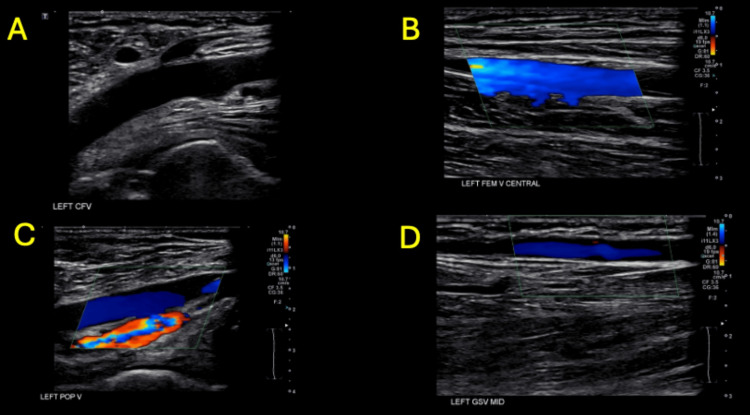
Left lower extremity venous duplex ultrasound There is no evidence of deep vein thrombosis or venous reflux within the left common femoral vein (CFV) (A), left femoral vein central (FEM V CENTRAL) (B), left popliteal vein (POP V) (C), and left middle great saphenous vein (GSV MID) (D).
